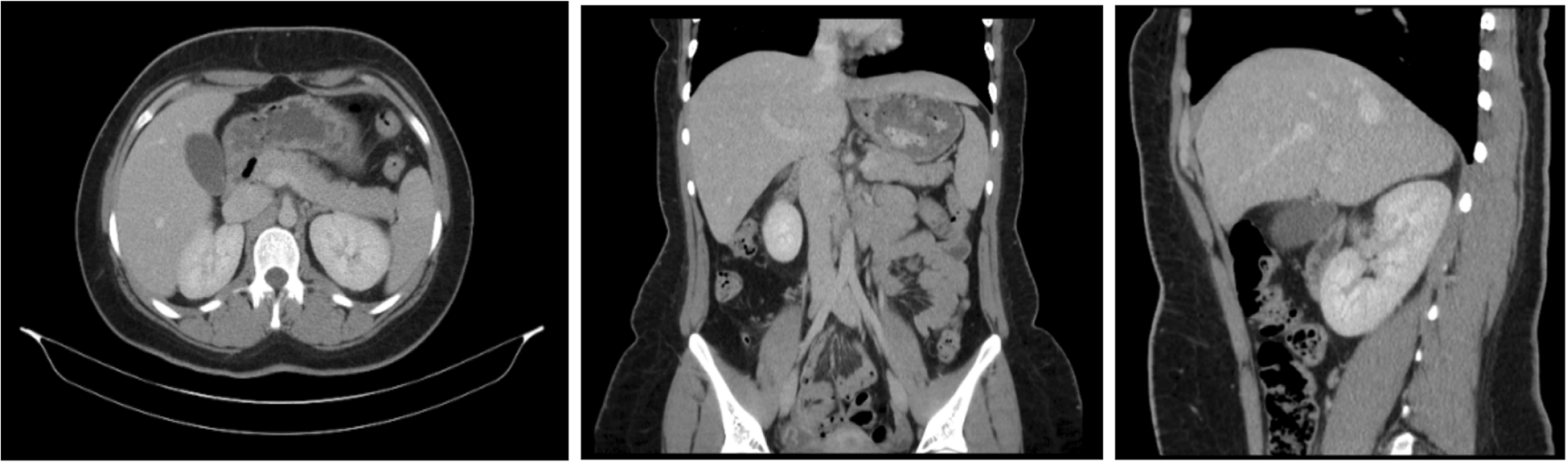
Non-contrast CT images of 19 y/o female in 2018 (a) axial (b) coronal (c) sagittal
